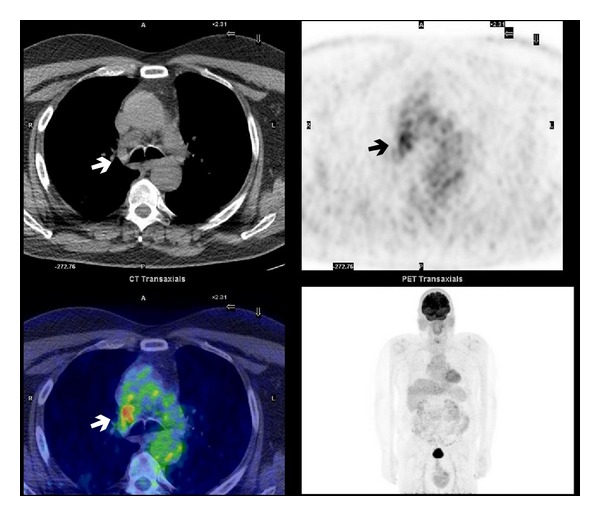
Right paratracheal suspicious lymphadenopathy at PET/CT scan—SUVmax 4.6 (arrow).
